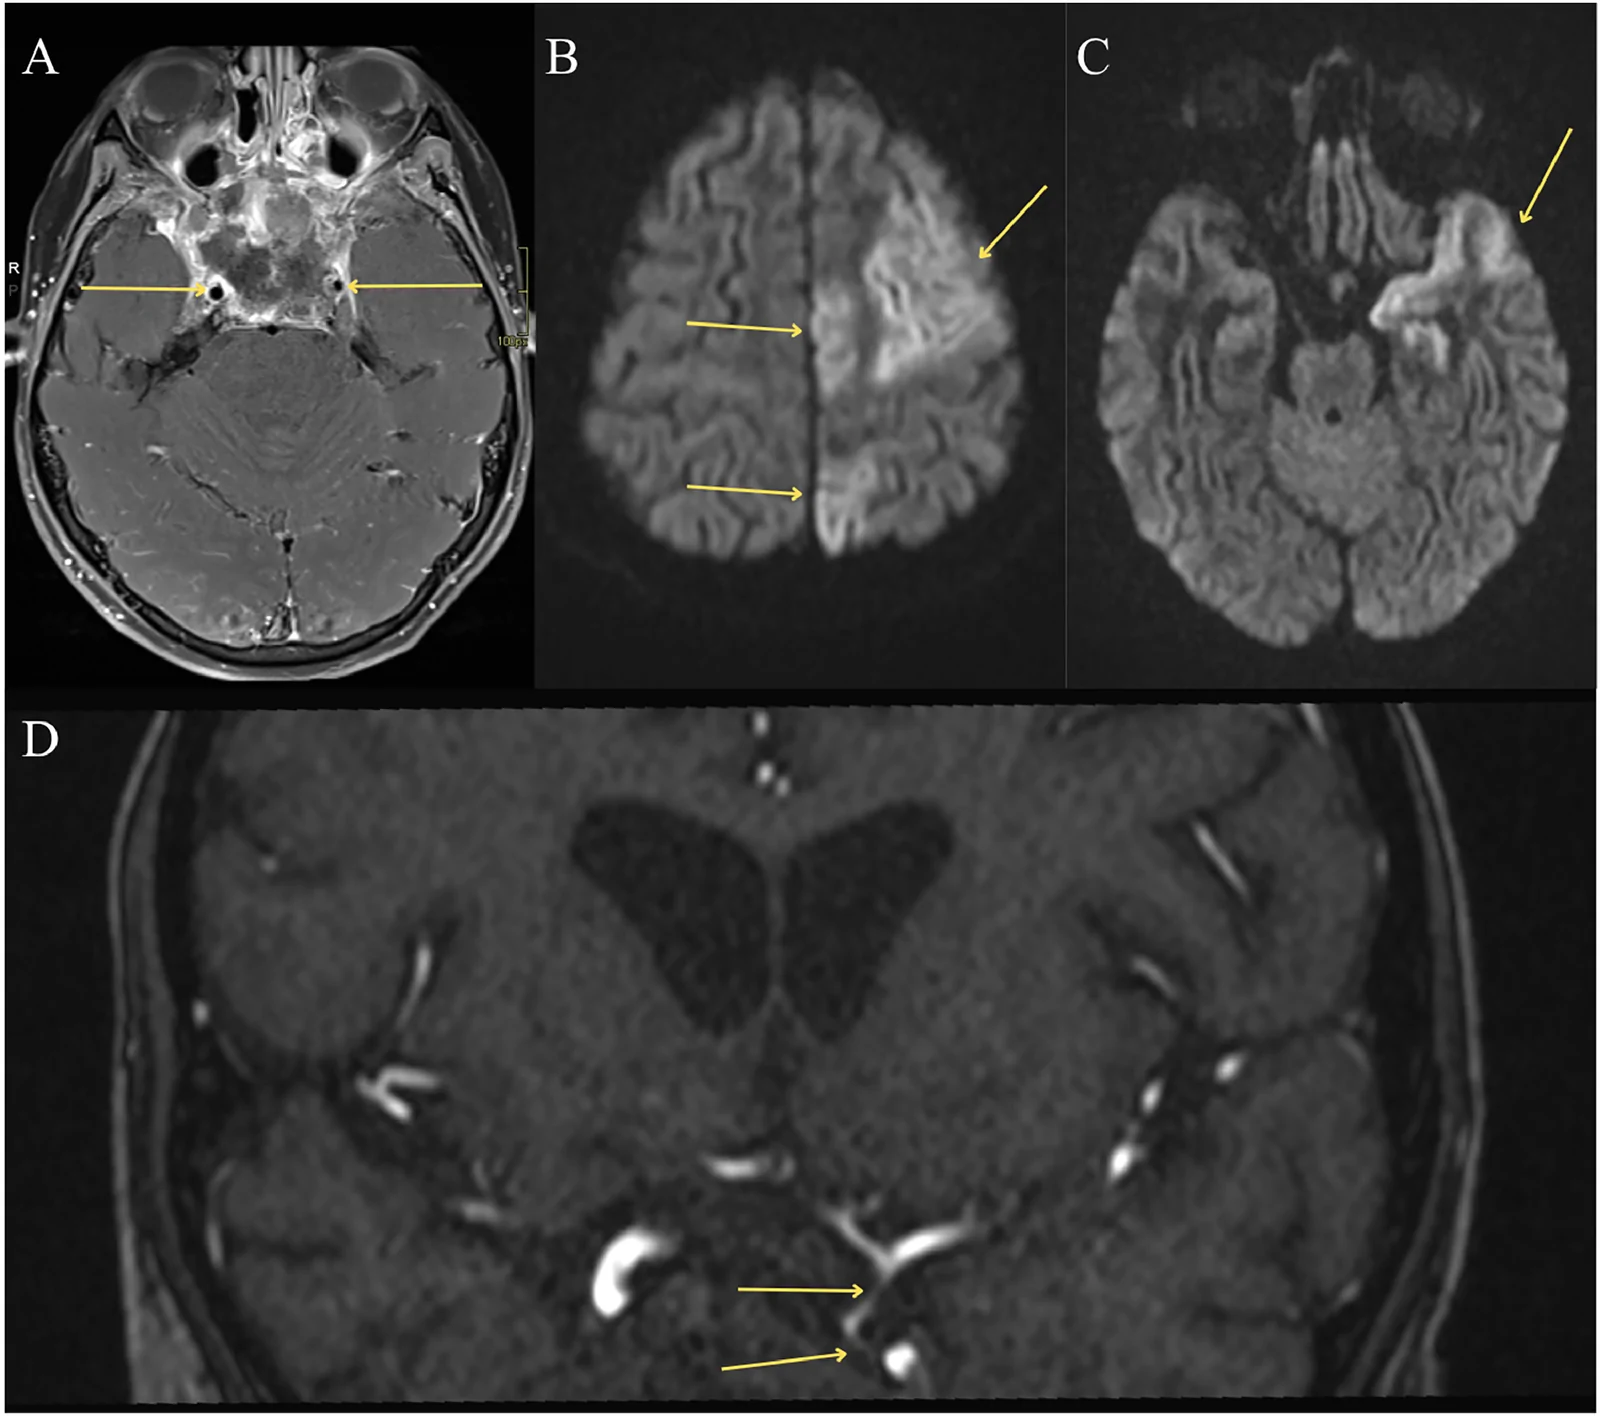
Vascular complications of SMARCB1-deficient sinonasal carcinoma. (A) Axial contrast-enhanced T1-weighted image demonstrates tumor encasing the bilateral internal carotid arteries (arrows), with asymmetric narrowing of the left internal carotid artery. (B) Diffusion-weighted imaging demonstrates acute infarction within the left cerebral hemisphere, predominantly involving the MCA territory with additional ACA watershed infarcts (arrows). (C) Diffusion-weighted imaging at a more inferior level demonstrates additional infarction involving the left anterior temporal lobe (arrow), consistent with an MCA infarct. (D) Subsequent MR angiography demonstrates severe narrowing to near-complete occlusion of the left internal carotid artery (arrows).
